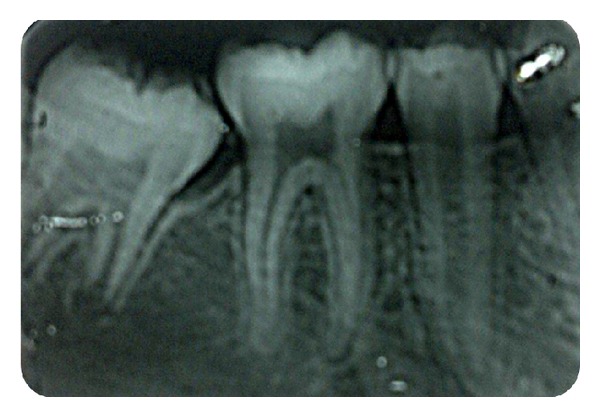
Intraoral periapical radiograph after 3 days with elastic placed.
